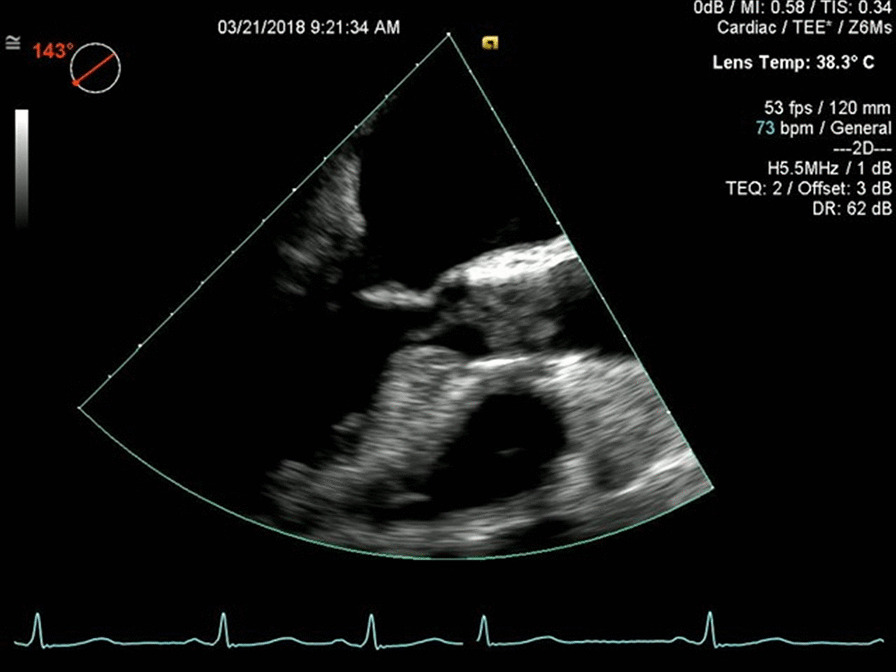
Preoperative trans-esophageal echocardiographic image showing obstruction to the valve orifice caused by the intraluminal growth of the infectious vegetation
